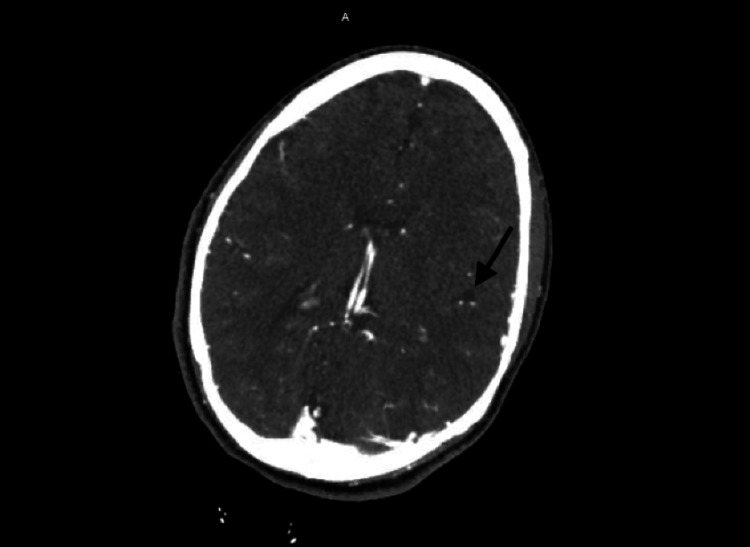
CT angiogram of brain showing occlusion of the M3 branch of the left middle cerebral artery in the left lateral frontal lobe
